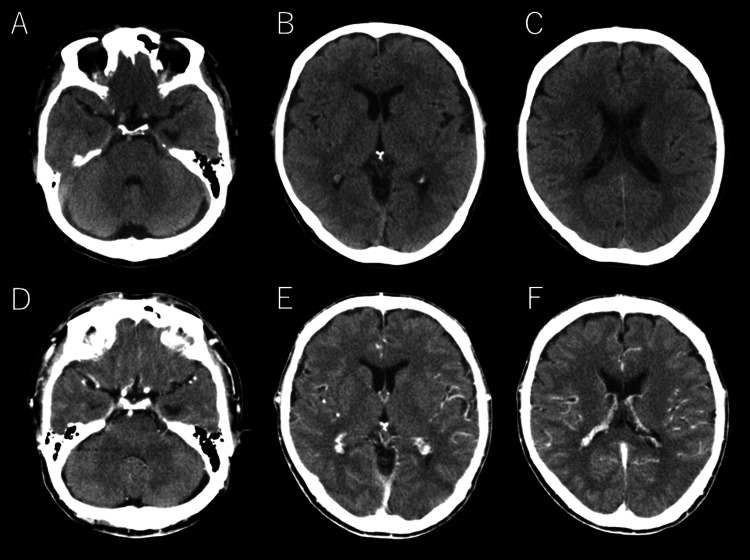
Head computed tomography results at admission Non-contrast head computed tomography (CT) (A-C) and contrast-enhanced CT (D-F) showed no evidence of intracranial hemorrhage or venous sinus thrombosis as the cause of the patient's headache.
